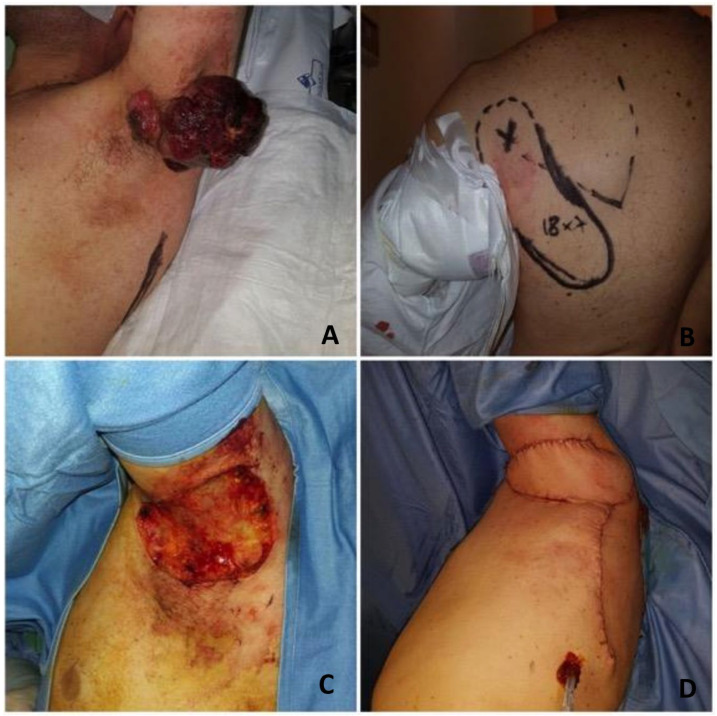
A 50-year-old man presented with a large exophytic ulcerated melanoma metastasis of the left axilla after the previous removal of cutaneous melanoma of the dorsum with positive sentinel node and axillary clearance refusal, 2 years before (A). The parascapular artery perforator was found with the handheld Doppler and the flap was marked preoperatively. (B) Palliative removal of the metastasis in continuity with the axillary lymphadenectomy was performed. (C) Strict adherence to the axillary vessels was present and the reconstruction of the medium-sized soft tissue defect of the axilla was achieved with a parascapular perforator-plus flap. (D) Medical treatment of the melanoma was started.
